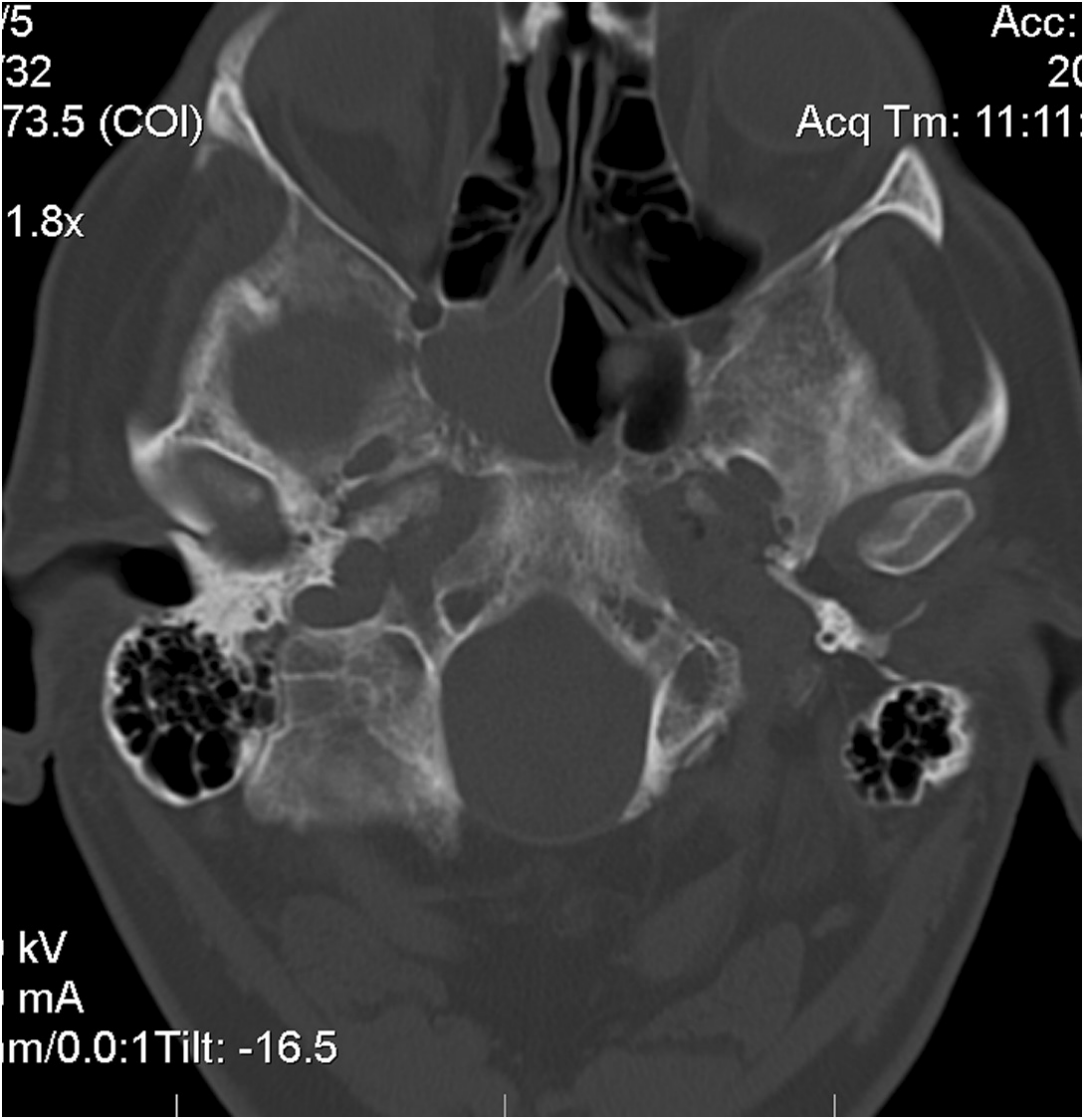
CT image of mass in sphenoid sinus transverse
